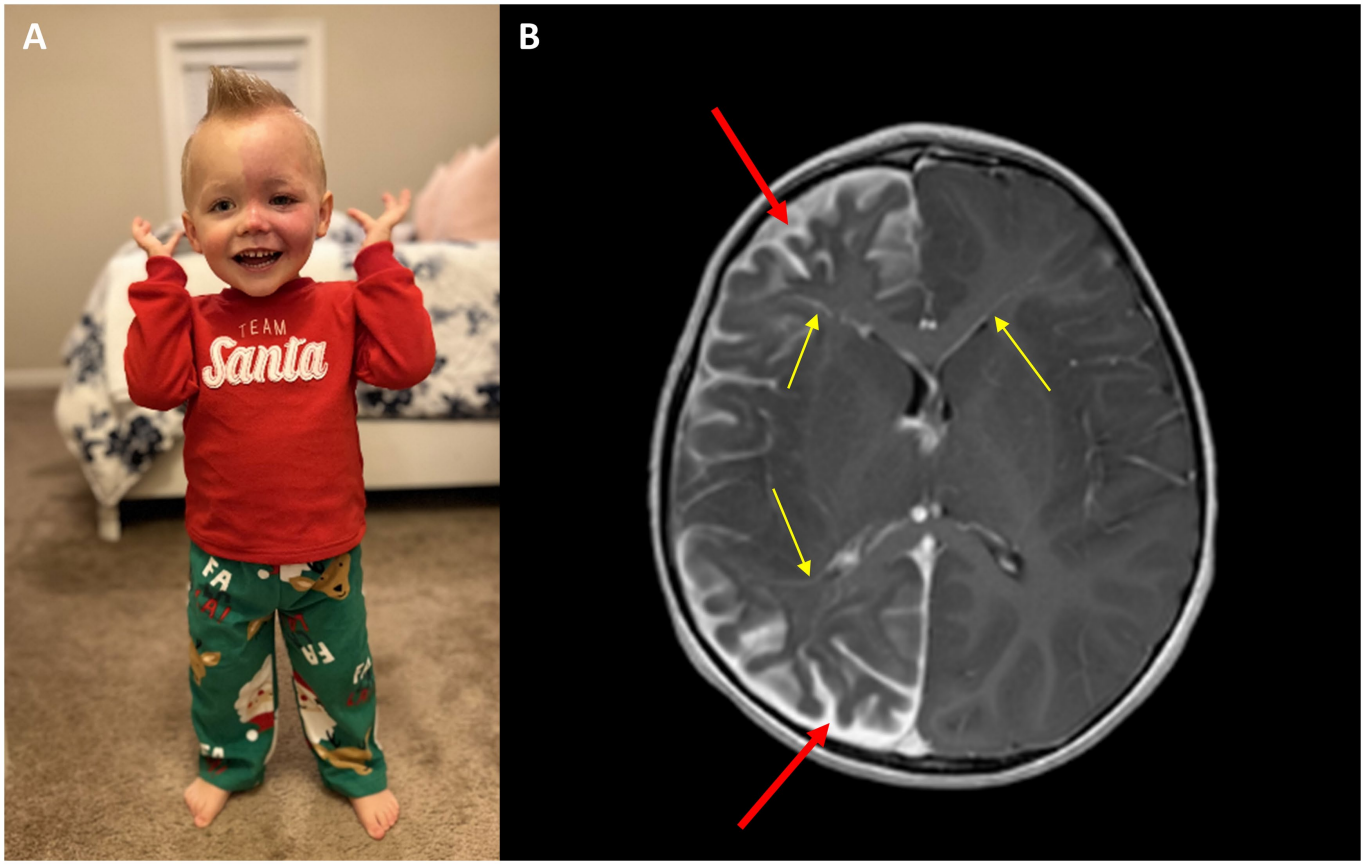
(A) Image of a SWS patient with a left facial port-wine birthmark and (B) corresponding T1 post-contrast MRI indicating leptomeningeal involvement and abnormal blood vessels, indicated by red arrows. Yellow arrows indicate enlarged deep veins that flow into the cortex.
